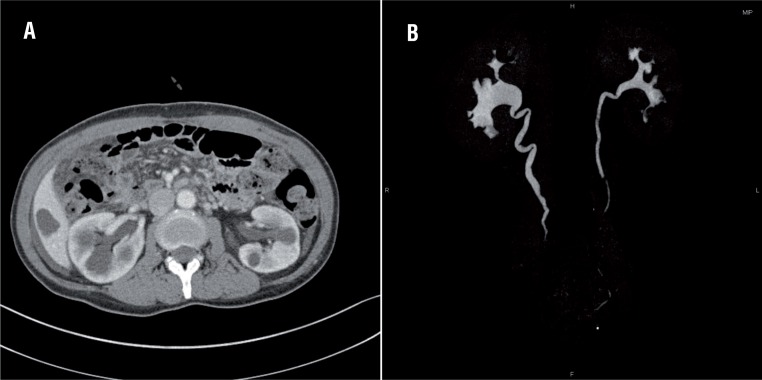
Post-operative contrast-enhanced abdominal computed tomography scan shows the partial improvement of hydronephrosis in the axial scan (A) and the coronal scan of urography (B).
